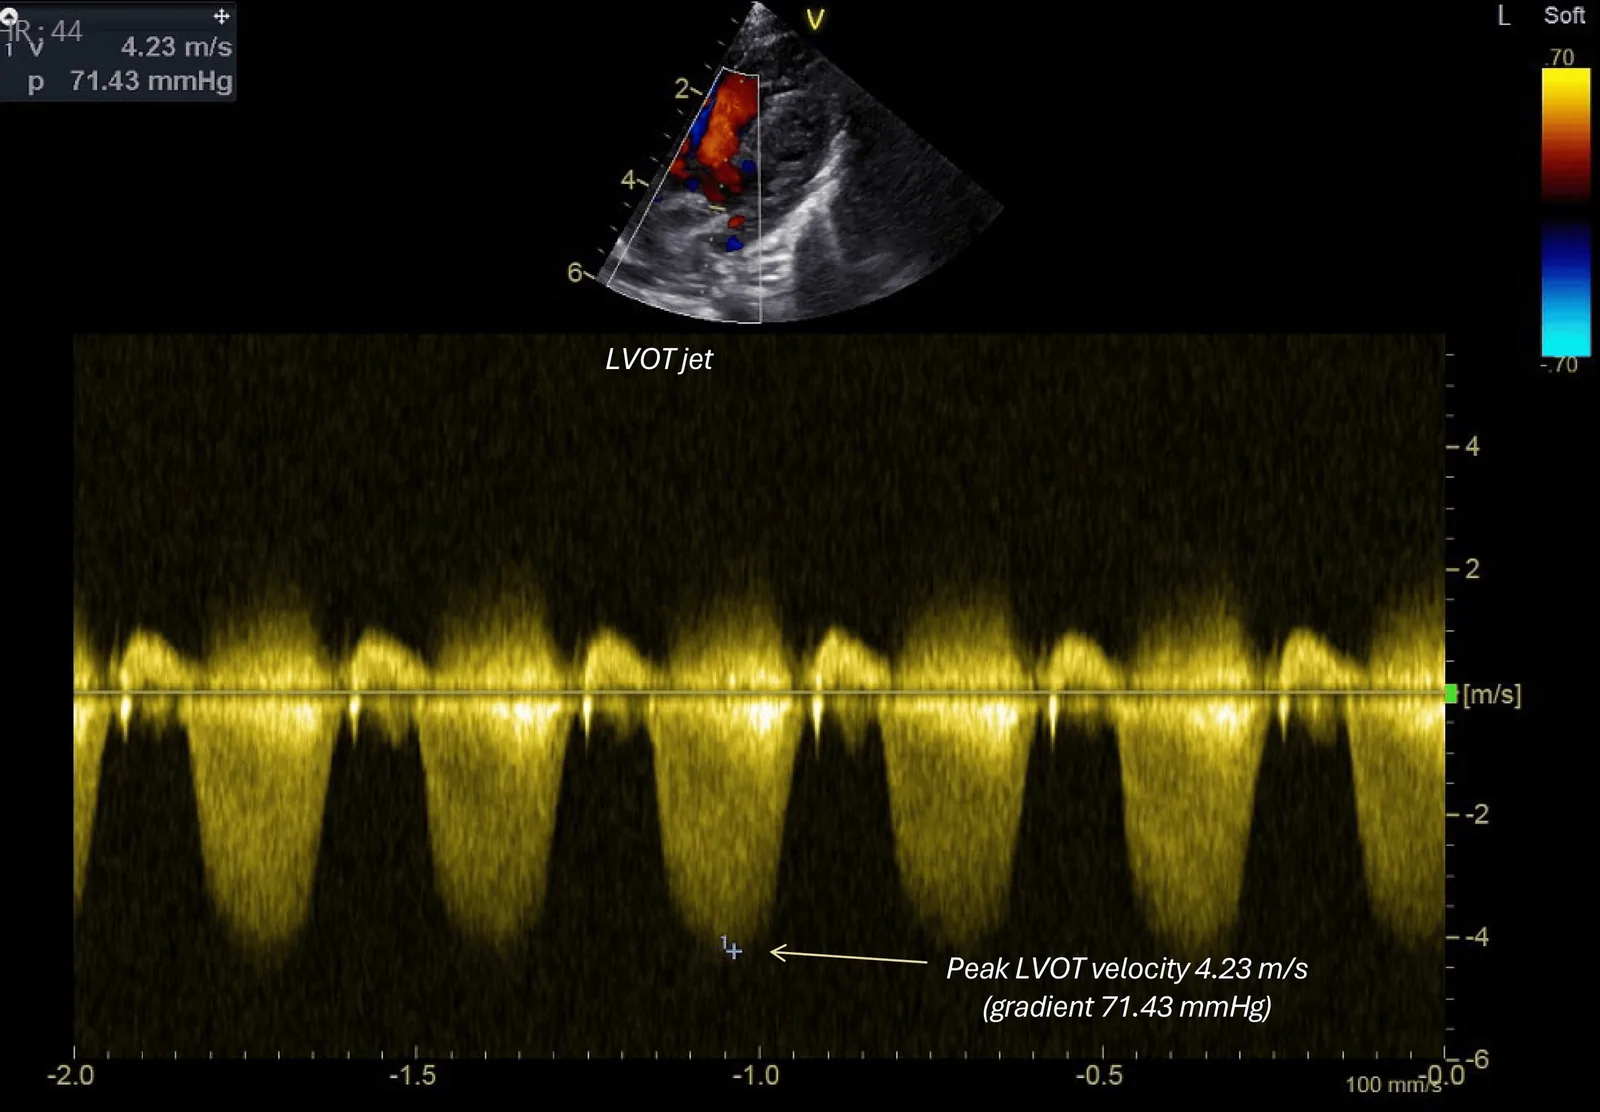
Preoperative continuous-wave Doppler across the left ventricular outflow tract. Spectral trace demonstrating a peak velocity of 4.23 m/s, corresponding to a peak instantaneous pressure gradient of 71.43 mmHg. LVOT, left ventricular outflow tract.
